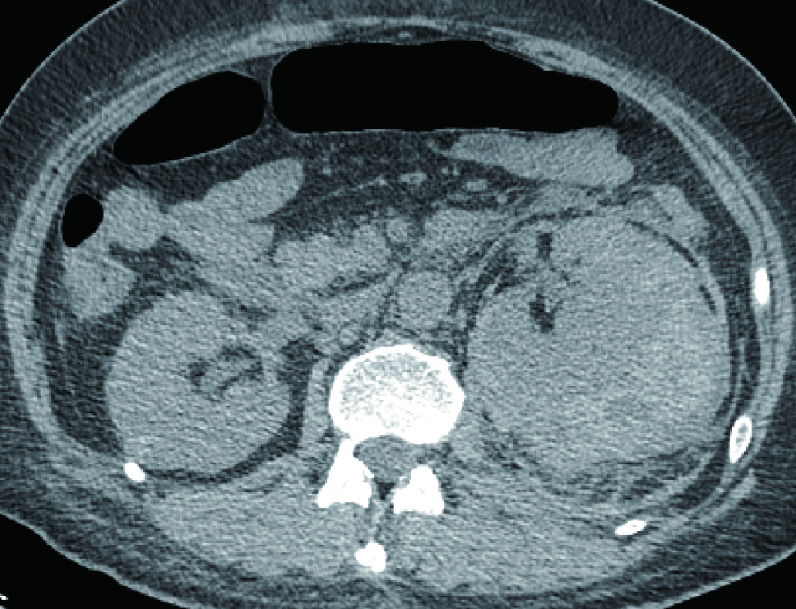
腹部CT示左侧肾筋膜内巨大血肿 CT scans of the abdomen shows large hematoma within the left renal fascia
